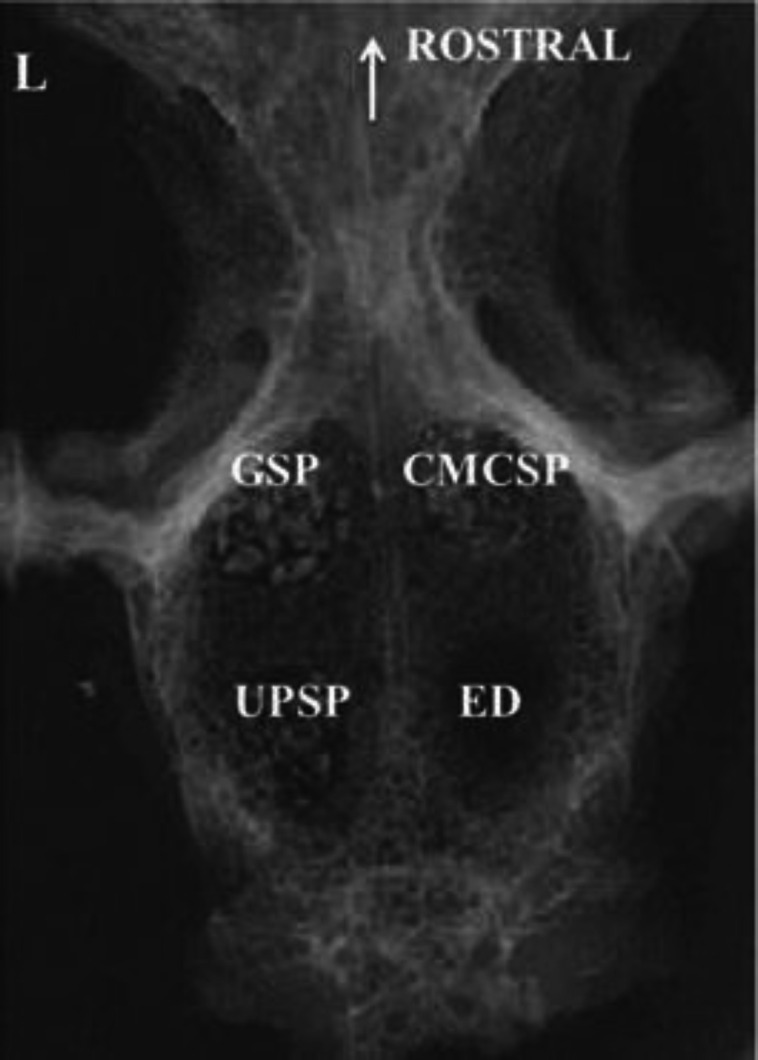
Post-operative dorsoventral radiographic view of the operated region. Arrow indicates rostral direction
